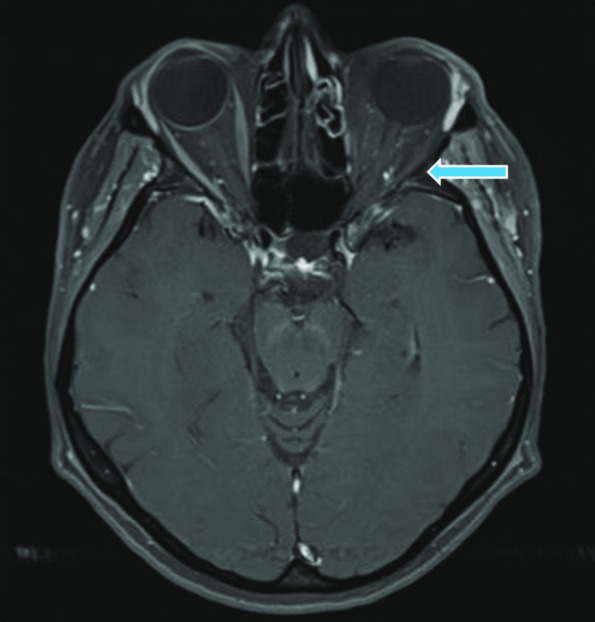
Post-contrast, axial T1-weighted fat-saturated image at presentation. The orbital structures, including the ocular bulb and the rectus muscles on the left side, show no contrast enhancement indicating an ischemic process
